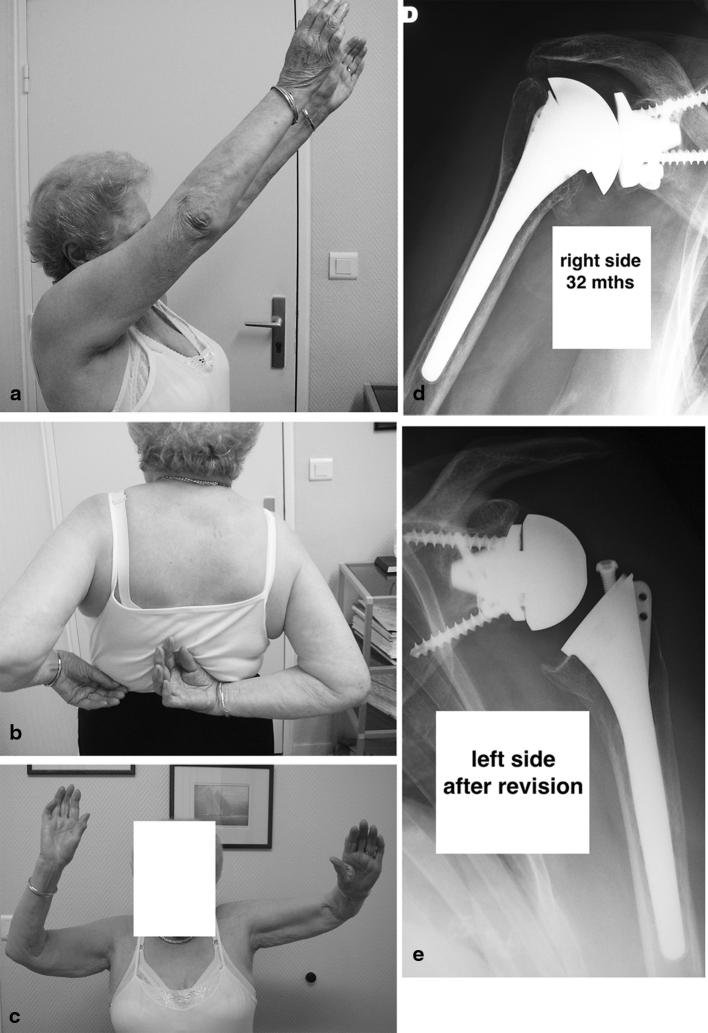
Patient operated on both sides with a metal back glenoid implant. No problem for the right side. On the left side a secondary cuff tear occured. A conversion for a reverse was realised with a good clinical result a–c clinical results at 36 months, d X-ray on the right side e X-ray on the left side after the revisionOne case sustained a superior migration due to a bad initial choice of the size of the glenoid with a too low implantation. This allowed a quick superior migration of the humeral head above the glenoid implant but without cuff tear. The revision at 1 month consisted on a higher implantation of a bigger metal tray. The MB 44 was converted to 48. This patient was 60 months of follow-up, and this case of revision was included in the final review.One patient had a postoperative painful stiffness because of a complex regional pain syndrome.Another case of painful shoulder was reoperated at 6 months. No aetiology was found. The MB glenoid was converted to a cemented glenoid. At 13 months, there was a superior migration with a bad result.
